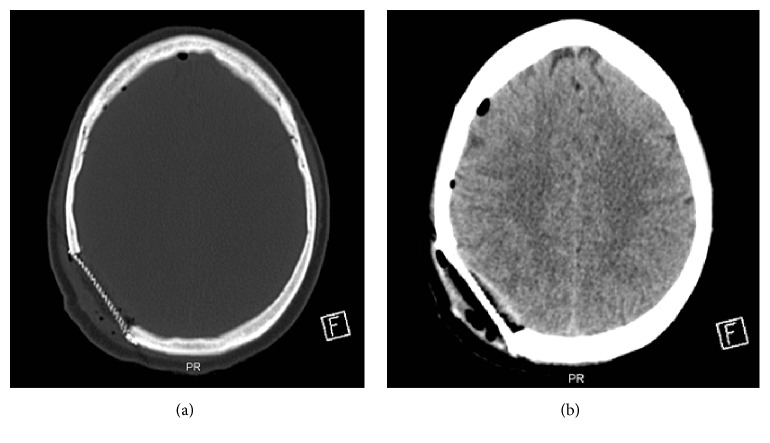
Postoperative nonenhanced (a) bone window and (b) brain window axial CT showing a metallic mesh over the site of bony resection, with no associated intracranial hemorrhage or residual bony lesion.
